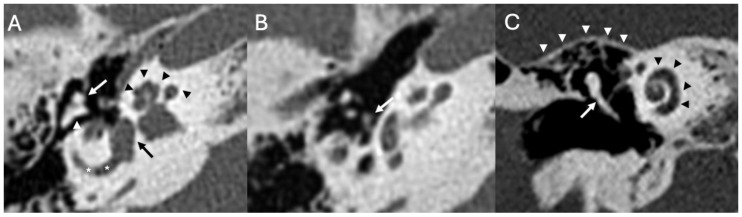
Axial (A,B) and coronal reformat CT (C) showing the ear ossicles, namely the malleus (white arrows in (A,C)), incus (white arrowhead in (A)), and the stapes (white arrow in (B)). The image also depicts the cochlea (black arrowheads in (A,C)), the vestibule (black arrow in (A)), the lateral semicircular canal (white asterisks in (A)) and the tegmen tympani (white arrowheads in (C)).
